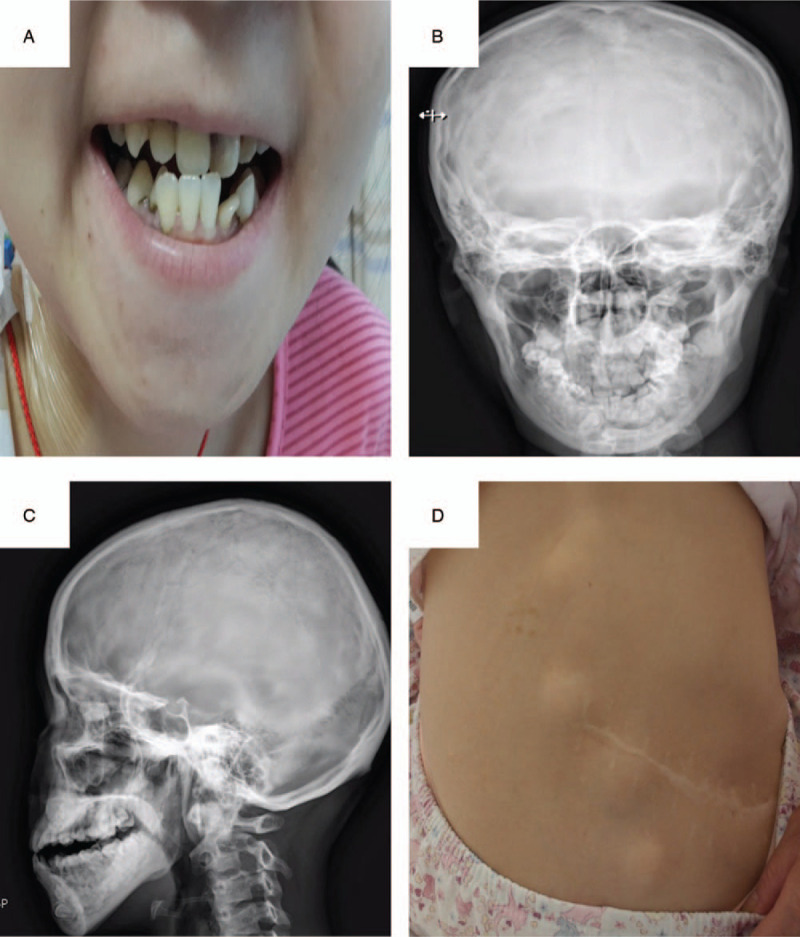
Dental abnormality of a patient with GS. (A) Initial intraoral clinical aspect of the patient. (B and C) Panoramic radiograph showing the presence of supernumerary teeth. (D) Photograph showing epidermoid cyst over the lower back.
